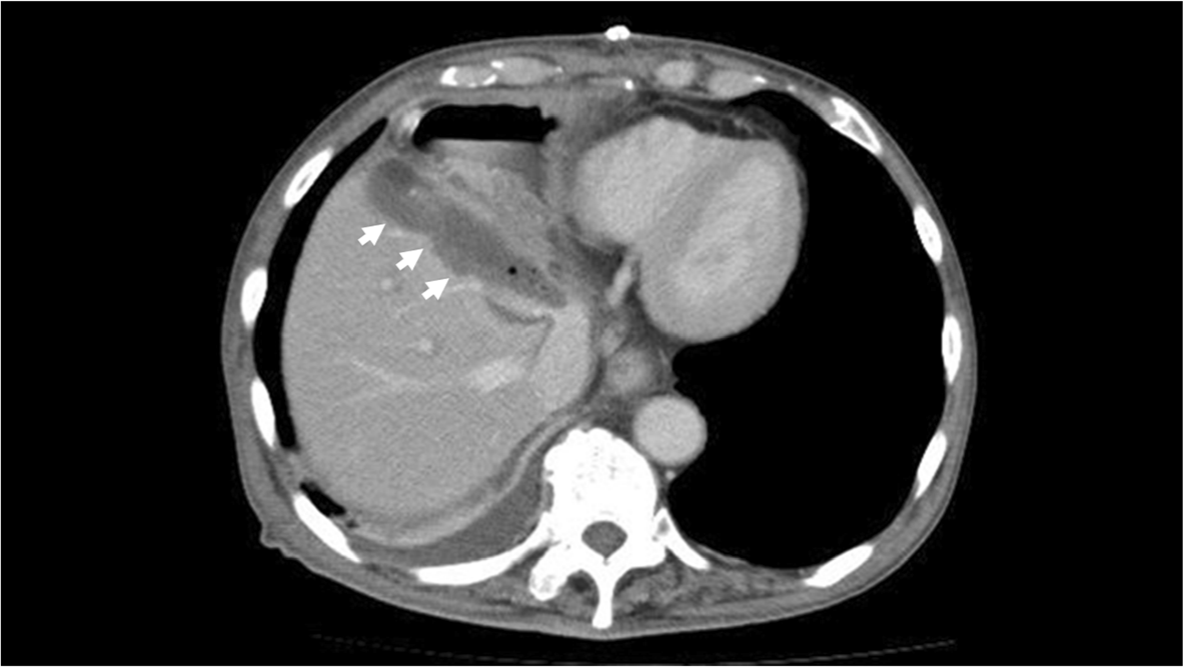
An enhanced abdominal CT scan showed fluid collection at the transected liver surface (arrow)
